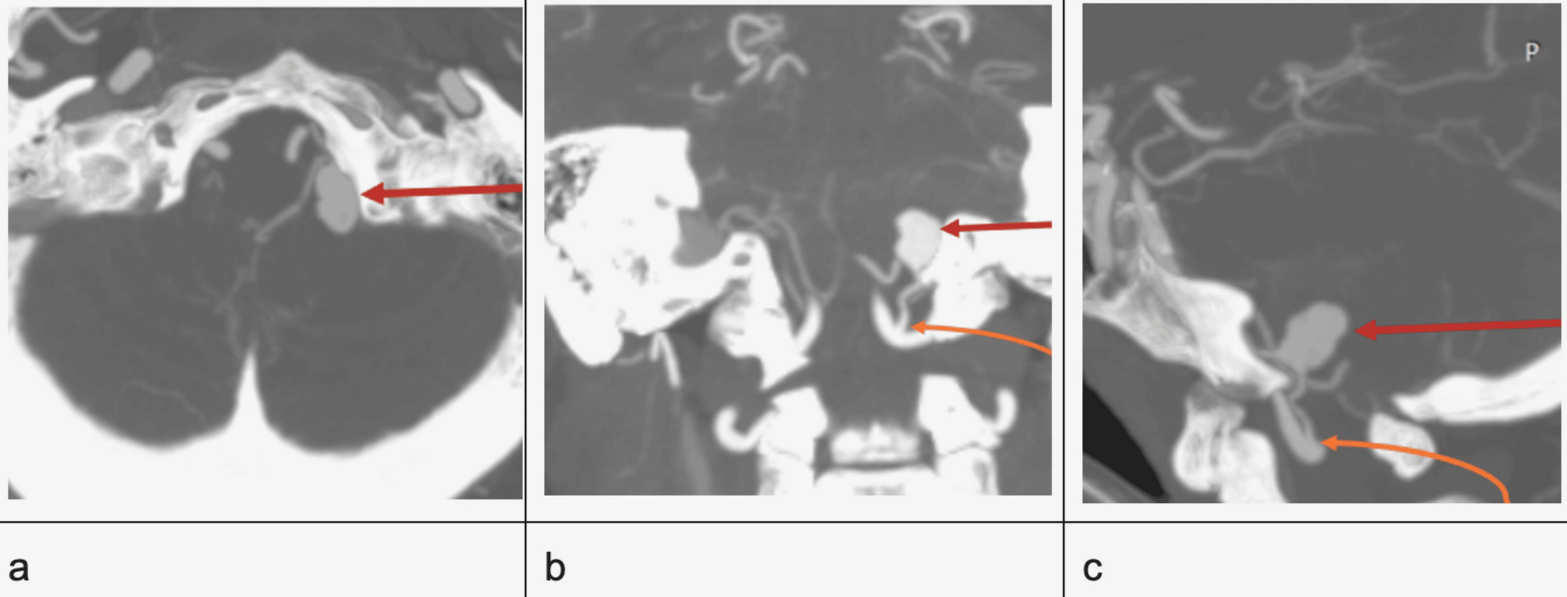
CT angiography in maximum intensity projection (MIP) reconstruction Wide neck saccular aneurysm (red arrows) in the left distal posterior inferior cerebellar artery arising from the left vertebral artery (orange arrows in b and c) seen in axial (A), coronal (B), and en-profile view on sagittal image.
